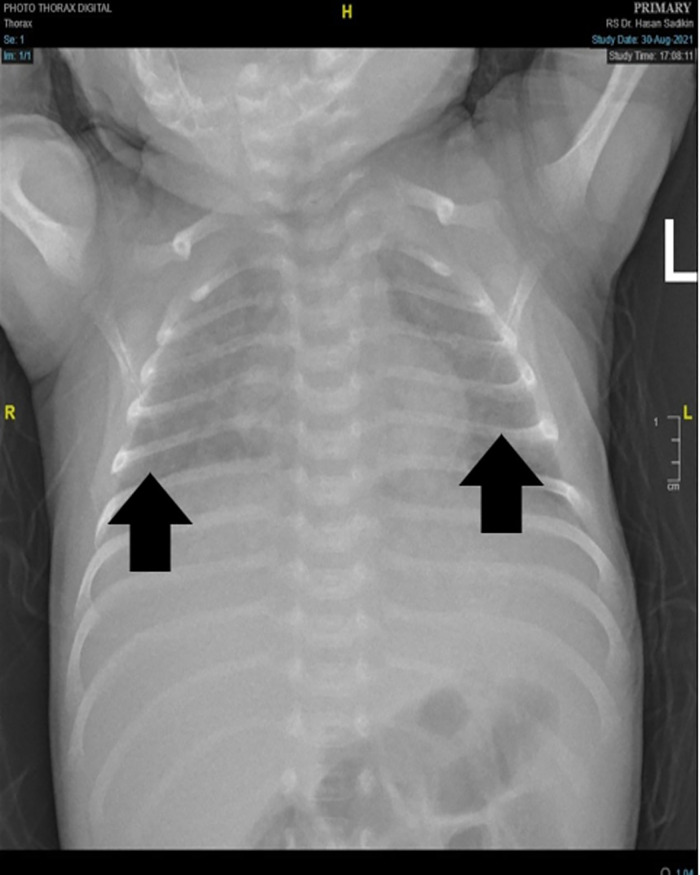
Chest x-Ray. showed inhomogeneous opaque coverings within upper and lower lung fields bilaterally (black arrow).
